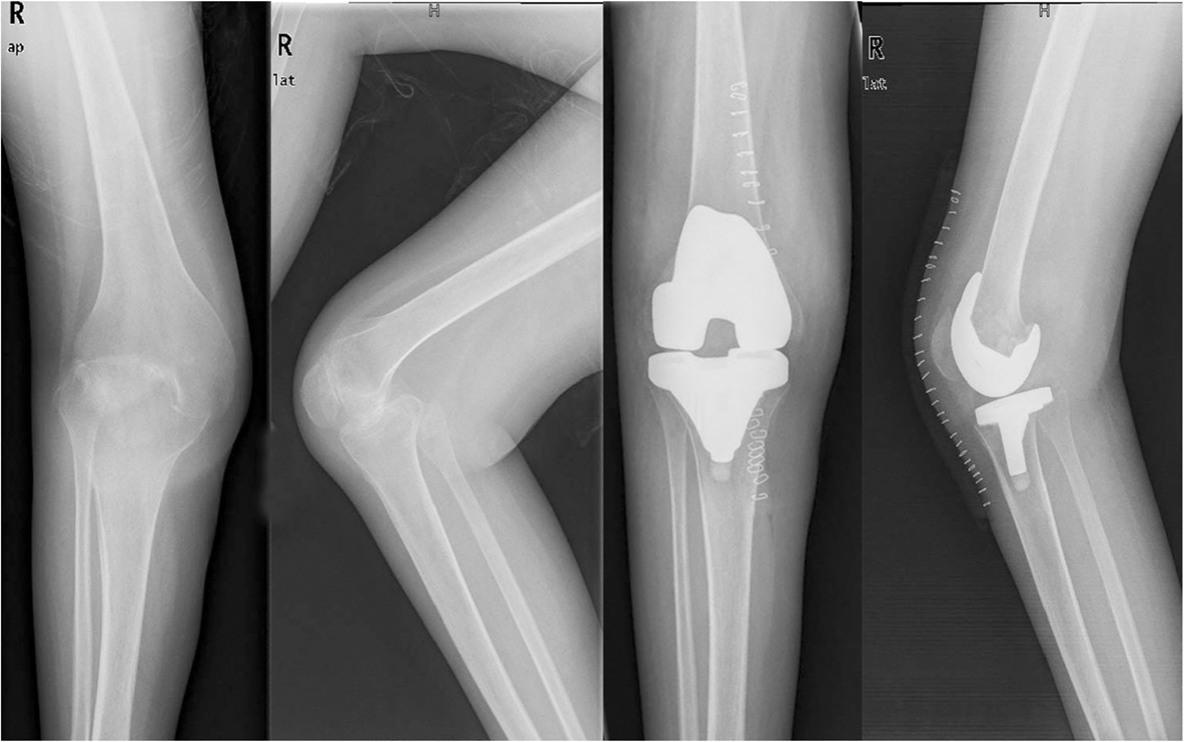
1 Plain anteroposterior and lateral radiographs of a 49-year-old female which reveal joint destruction. This case is a 49–year-old female patient with RA with flexion contractures deformity. After TKA on right knee, the 53° flexion contracture was completely corrected postoperatively.
